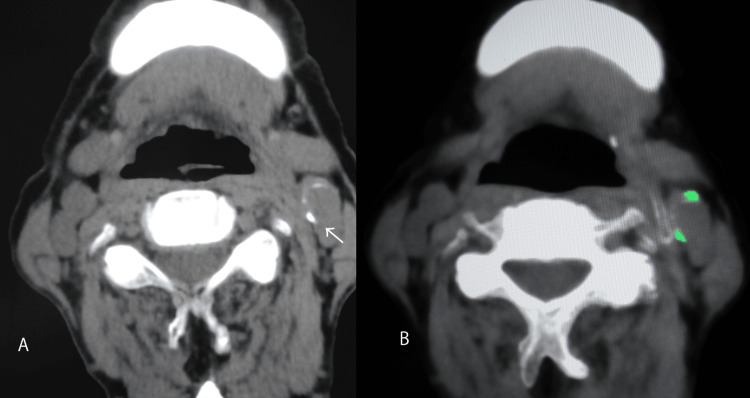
A representative case A 76-year-old man presented suddenly with left middle cerebral artery occlusion with cardiogenic embolism. The white arrow indicated a calcified plaque that was found in the left carotid artery (A). Dual-energy computed tomography (DECT) analysis of the calcified plaque showed that most of them were equivalent to urate indicated by light green (B).
